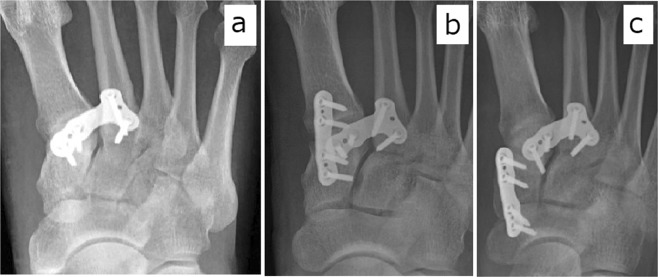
Postoperative radiographs of longitudinal type injury (a), transverse type injury combined with first tarsometatarsal joint injury (b), and Longitudinal injury extending into the naviculo-first cuneiform joint (c).
